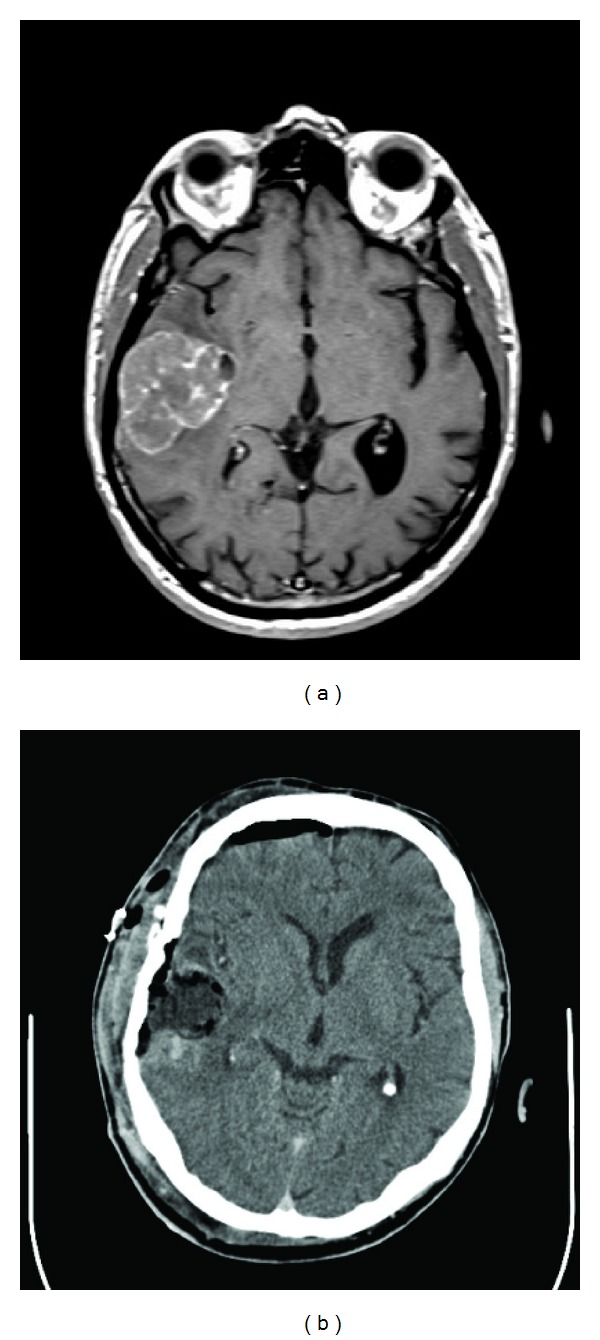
(a) Preoperative T1 axial contrast MR images showing a right temporal lesion with surrounded edema. (b) Postoperative head CT scan showing gross-total resection of the lesion.
